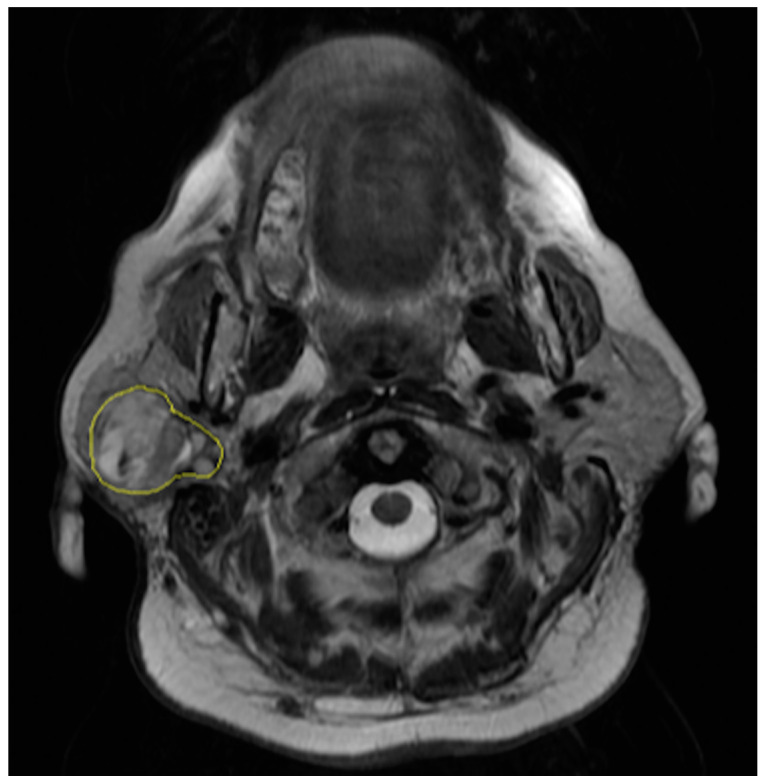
Segmentation of axial T2-weighted magnetic resonance (MR) image of a 61-year-old woman with a pleomorphic adenoma of the right parotid gland.
